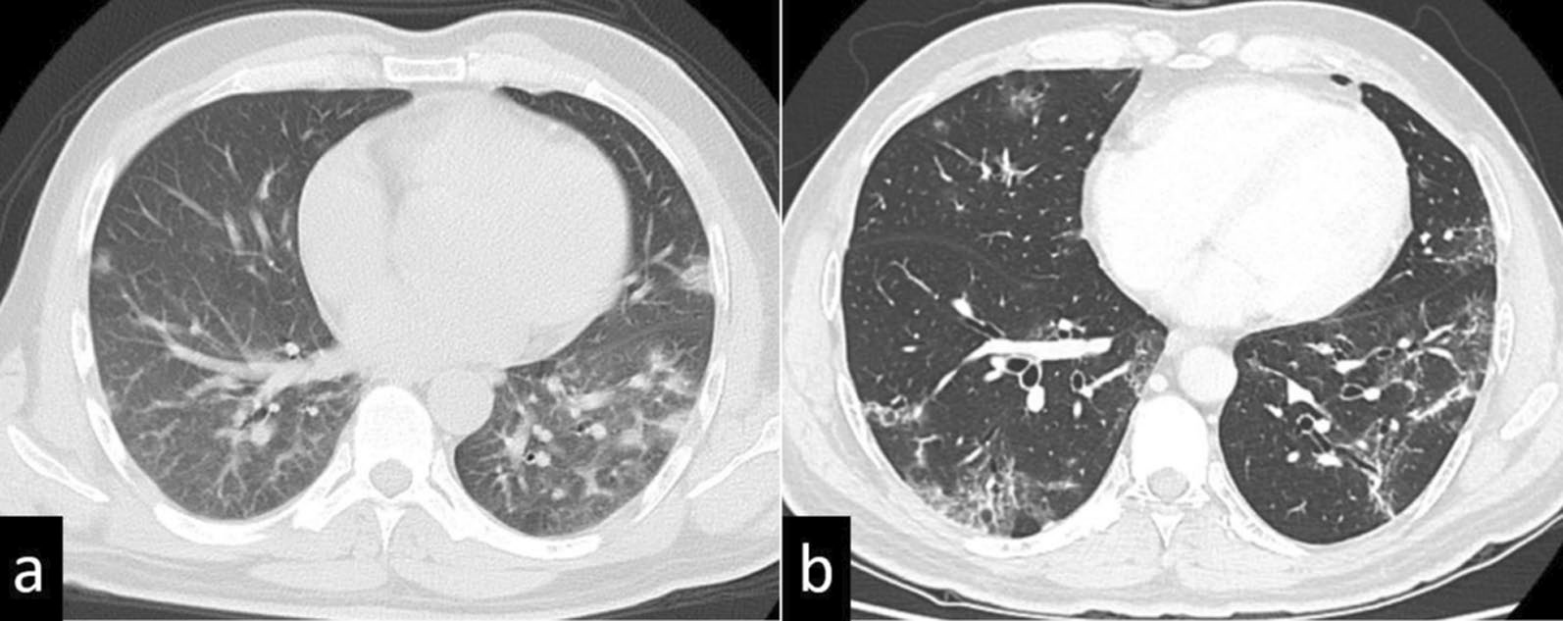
Findings of case 3 with chest computed tomography. a Ground-glass opacities and faint shadows were identified in bilateral lower lobes immediately after COVID-19 infection. b The opacities and shadows had regressed 19 days before the operation
